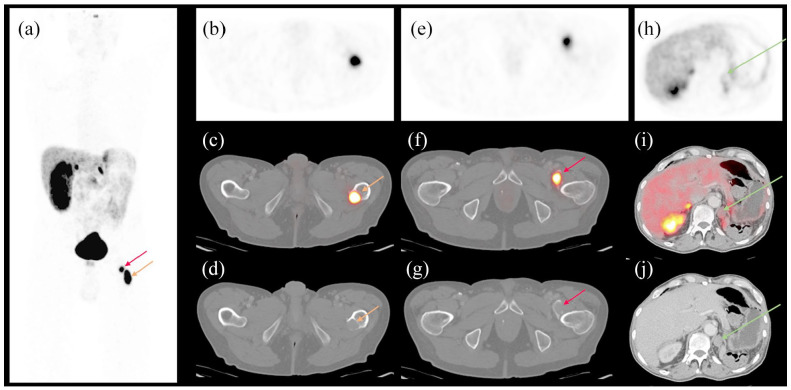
DOTATATE PET/CT 1 month after presentation. (a) Maximal intensity projection view. (b–d) Axial view of the remaining lytic lesion of the lesser trochanter of left femur (red arrows). (e–g) Axial view of the detached lytic lesion from the lesser trochanter of left femur (yellow arrows). (h–j) Axial view of the left adrenal nodule (green arrows). (b, e, and h) DOTATATE PET images. (c, f, and i) Overlay of PET and CT images. (d, g, and j) CT images.
